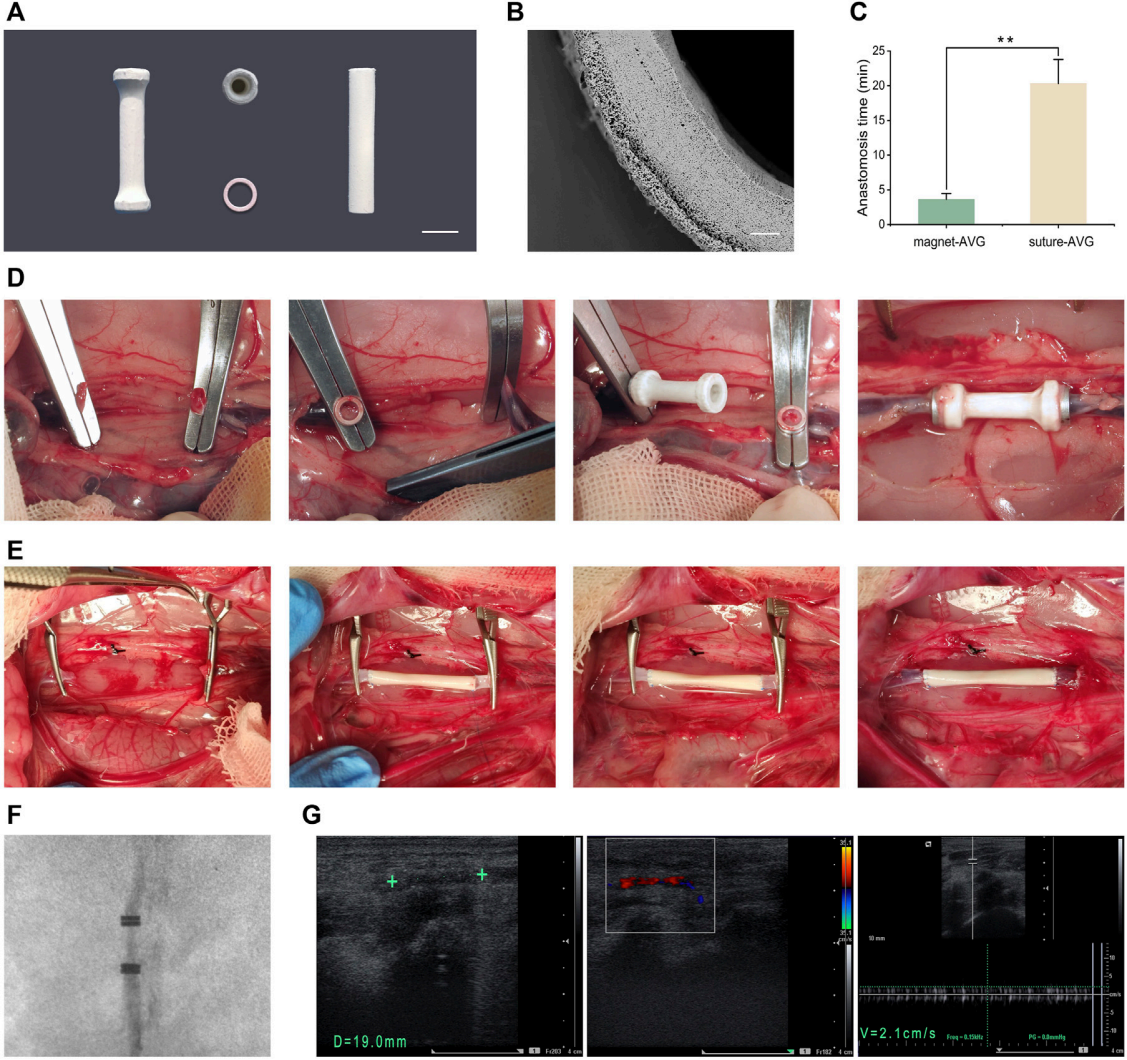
In vivo* implantation. (A) Images of mag-AVG, magnetic rings and suture-AVG; (B) The cross-sectional SEM image of AVG; (C) Comparison of the anastomosis time between mag-AVG and suture-AVG. (D) Images of mag-AVG implantation; (E) Images of suture-AVG implantation. (F) X-ray image of the implanted mag-AVG; (G) Ultrasound images of the implanted mag-AVG on the 2nd day post-implantation; Measurement of AVG length (19 mm) and diameter (3 mm), “+” symbol representing the magnetic rings; Doppler ultrasound reveals blood flow signals within the AVG; The blood flow velocity within the AVG is recorded at 2.1 cm/s.
